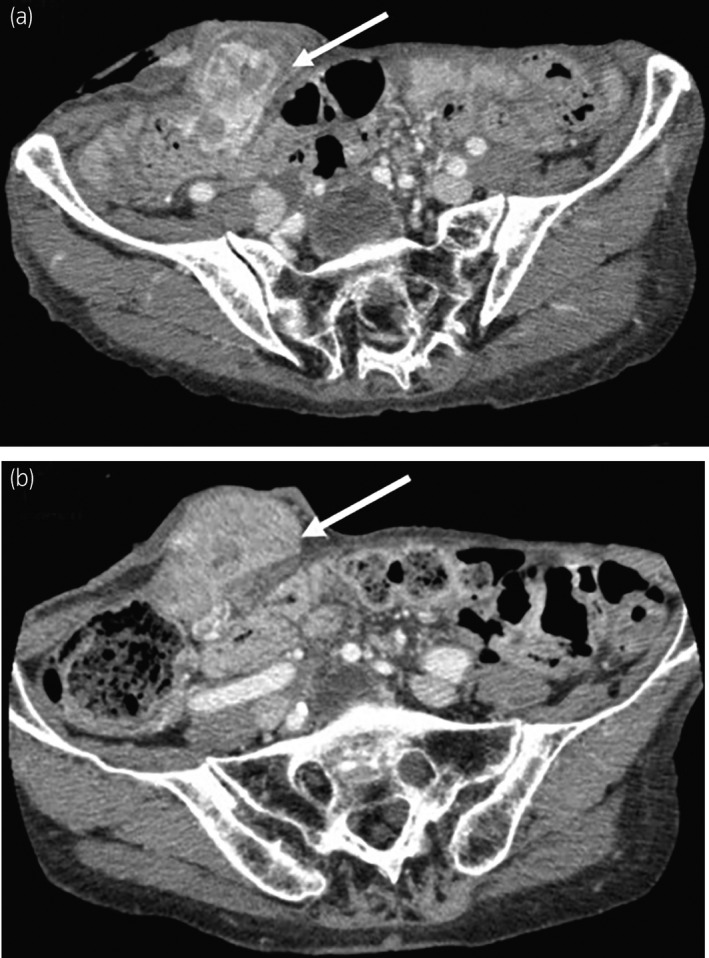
A white arrow points to: (a) a 35 × 30 × 25 mm mass detected by enhanced CT on the first admission with bleeding; and (b) a 50 × 45 × 40 mm mass detected by enhanced CT 3 years later.
